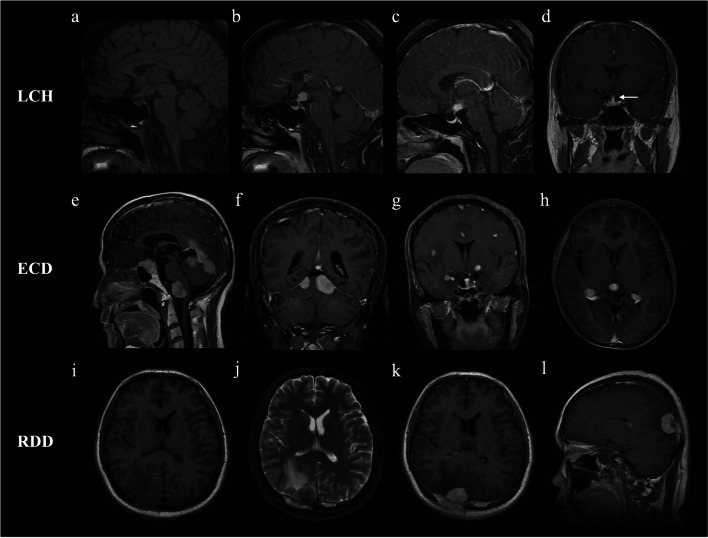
Representative cases of LCH, ECD, and RDD with tumorous lesions in the central nervous system. a, b A 41-year-old man with diabetes insipidus. T1-weighted image (WI) shows the loss of the posterior pituitary bright spot (a), with a single mass in the hypothalamus and infundibulum on enhanced T1WI b. c Enhanced T1WI of a 21-year-old woman with diabetes insipidus and hypogonadism shows the hypothalamic mass and pituitary atrophy. d Thickened pituitary stalk (arrow) was observed in a 21-year-old man with diabetes insipidus. e–f Enhanced T1WIs of a 50-year-old man with diabetes insipidus show multiple mass lesions in the saddle area and meninges. g–h Enhanced T1WIs of a 49-year-old woman show multiple tumorous lesions in the hypothalamus, infundibulum, and parenchyma with peritumoral edema. Tumorous lesions also appeared in the pineal gland and choroid plexus. i–l Classic imaging manifestations in a 33-year-old RDD patient include meningioma-like mass lesions in the bilateral occipital lobes, peritumoral edema, marked enhancement, and dural tail sign
